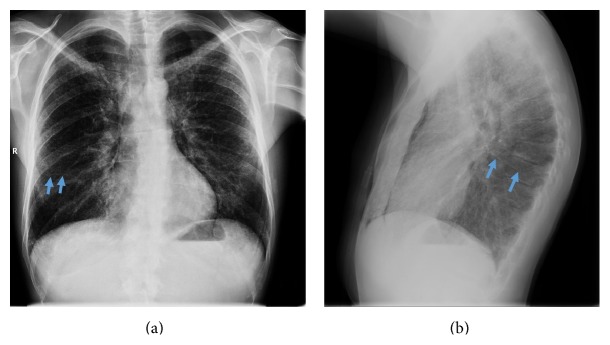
Plain X-rays. (a) anteroposterior X-ray; (b) lateral X-ray. Blue arrows show the superior accessory fissures (R = right side).
